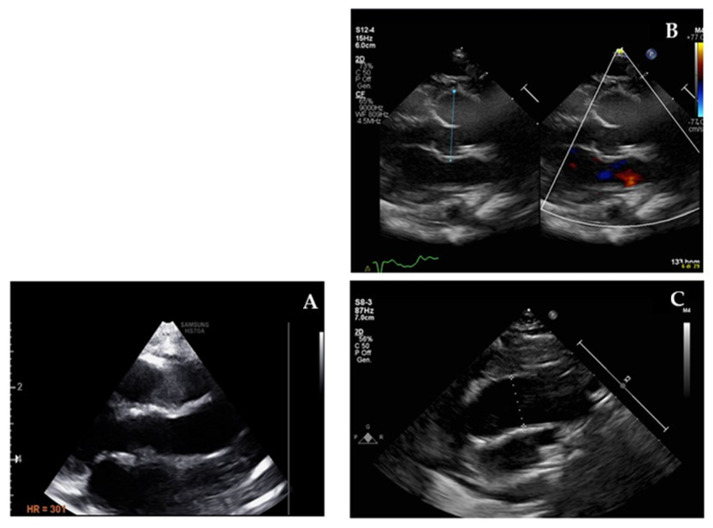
Echocardiographic imaging of normal ascending aorta (A) compared with patient 6 dilated aortic root (B) and dilated ascending aorta (C).
